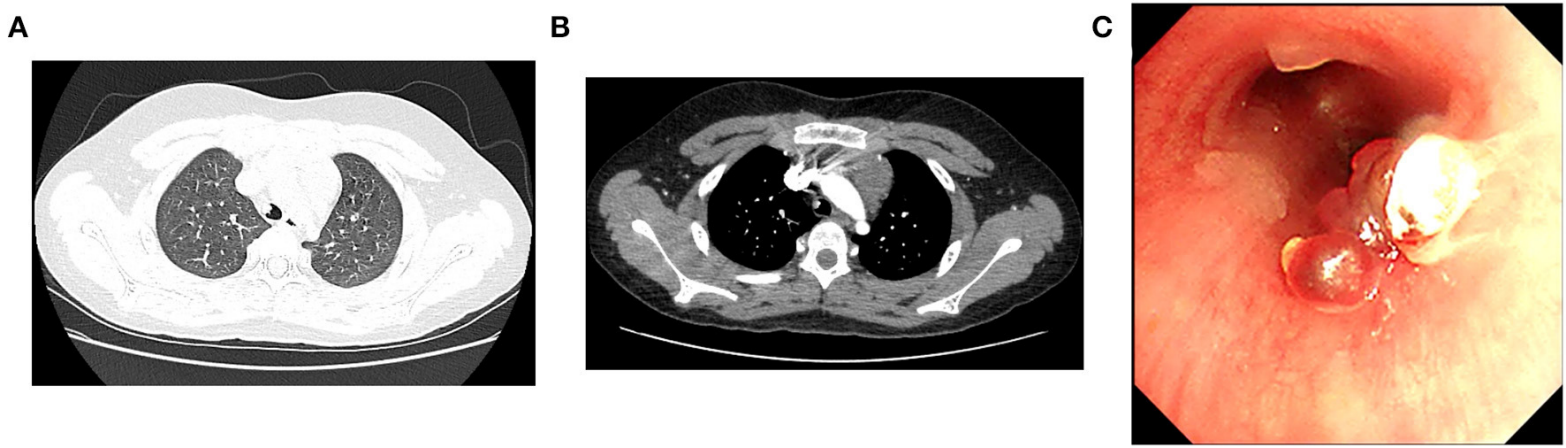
Both lung views of plain chest computed tomography (CT) scan (A) and mediastinal view of contrast-enhanced CT scan (B) demonstrate the tracheal mass. (C) Initial bronchoscopic view of the mass occluding the lower trachea.
